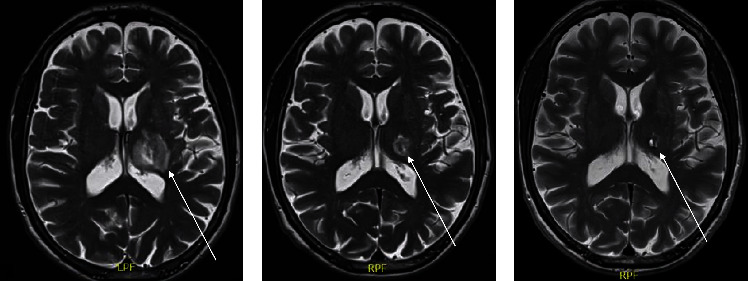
MRI imaging (T2 images, sagittal view) at presentation (a) and during follow-up (b) 5 weeks of TMP-SFX twice daily; (c) 20 weeks follow-up, of which 6 weeks of TMP-SFX 4 times a day and 14 weeks twice daily. Gradual improvement of the lesion was seen during treatment (highlighted by arrow).
